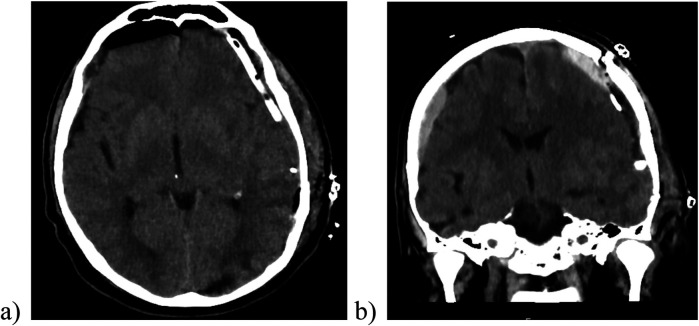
The postoperative cranial CT scan demonstrating a sufficient evacuation of hematoma, the placement of drain (image a) and ICP transducer in the subdural space (image b).
